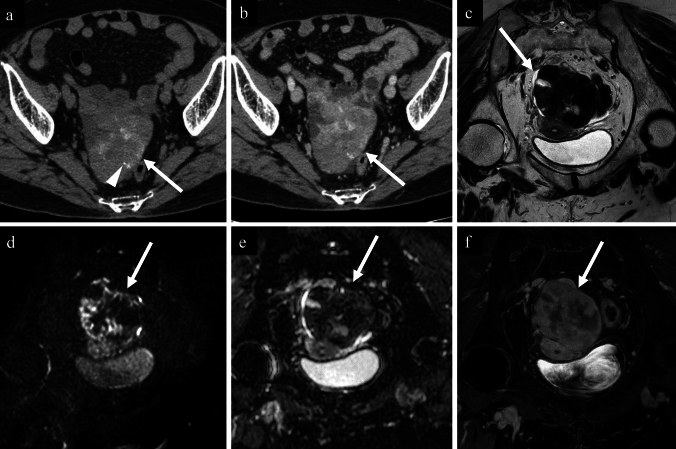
77-year-old woman with a Brenner tumour of the right ovary. a non-contrast CT; b contrast-enhanced CT; c coronal T2-weighted image; d coronal diffusion-weighted image; e coronal apparent diffusion coefficient map; f coronal contrast-enhanced T1-weighted image. A lobulated tumour with amorphous calcifications (a; arrow) and punctate calcifications (a; white arrowhead) is observed in the right ovary. Contrast-enhanced CT shows weak enhancement throughout the tumour (b; arrow), making the identification of calcification difficult on contrast-enhanced CT alone. This solid mass is heterogeneous but markedly low signals on T2-weighted image (c; arrow) with poor diffusion restriction on diffusion-weighted image (d and e; arrows). After contrast administration, enhancement is predominant at the periphery (f; arrow). The tumour was excised and diagnosed as a Brenner tumour
